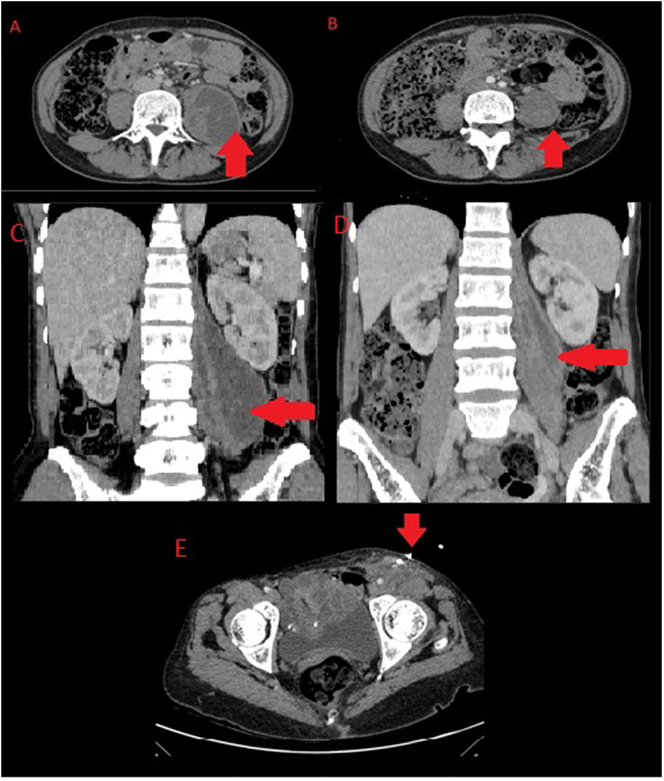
CT abdomen. A – Psoas abscess prior to drainage (Axial Image). B – Residual fluid 10 days post insertion of pigtail catheter (Axial Image). C – Psoas abscess prior to drainage (Coronal Image). D – Residual fluid 10 days post insertion of pigtail catheter (Coronal Image). E – Pigtail in situ.
